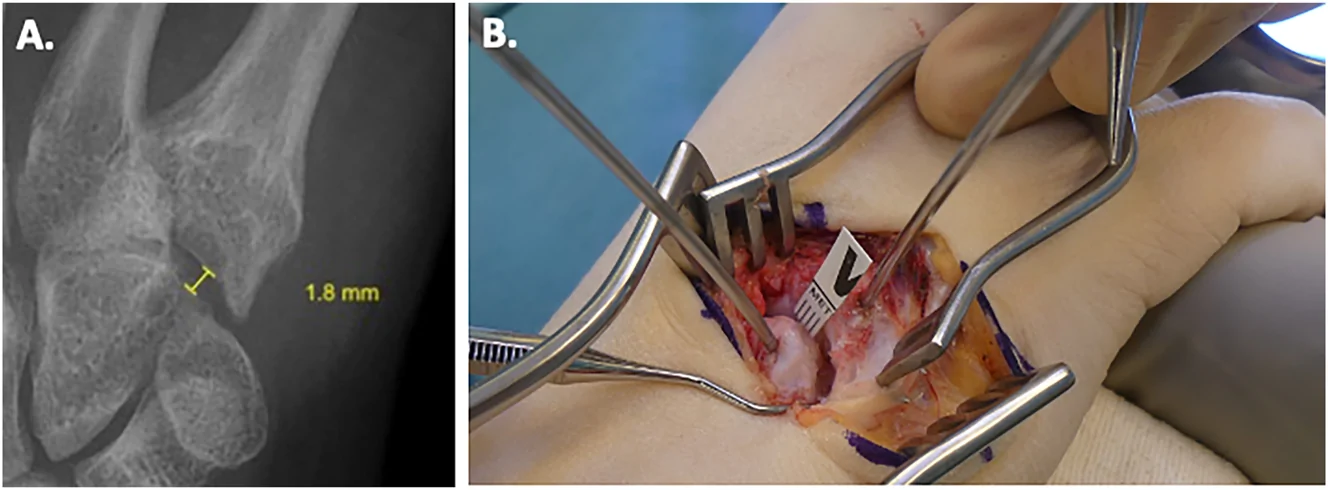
Preoperative imaging and intraoperative findings. (A) Preoperative X-ray image showing post-traumatic CMC-V arthrosis (joint space measured at 1.8 mm). (B) Intraoperative view of CMC-V osteoarthritis with central grade IV cartilage damage and osteophytic attachments.
